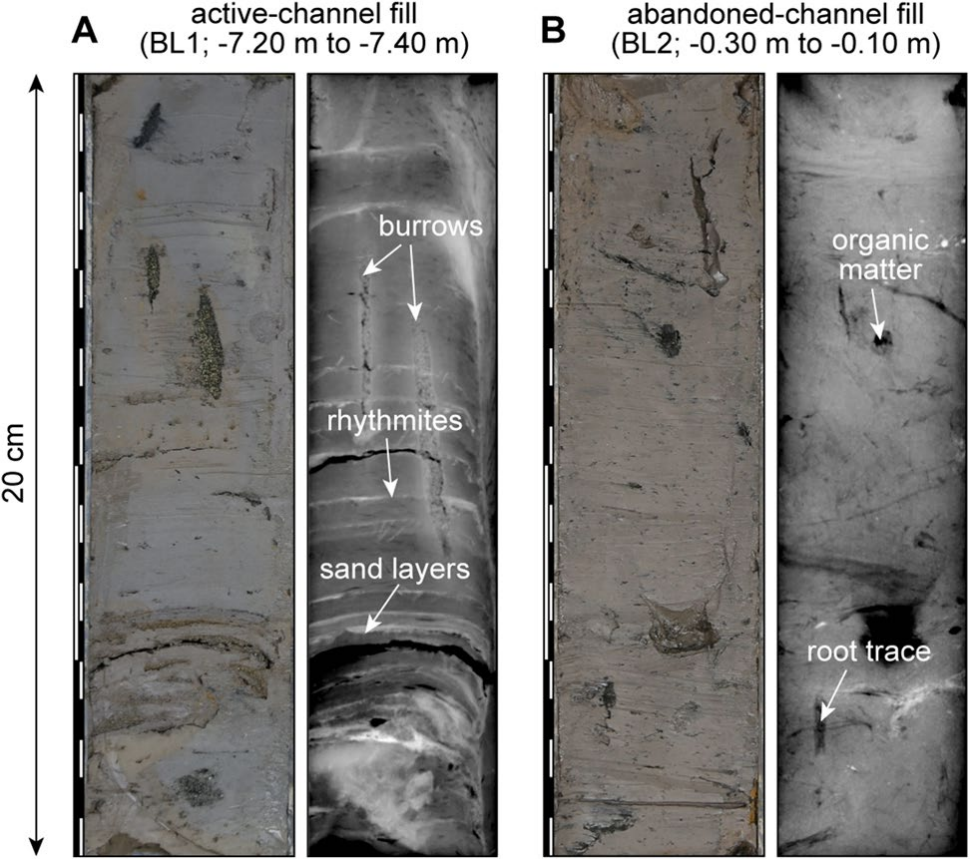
Selected photographs and x-radiographs showing a 20-cm-long part of the cores and highlighting the difference in sedimentary facies between the (A) active-channel fill and (B) abandoned-channel fill.
